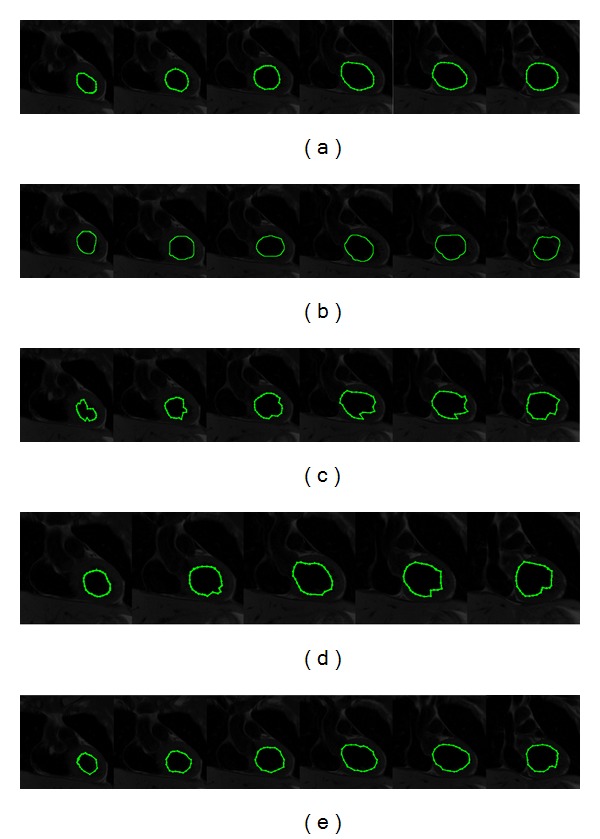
MR images (ventricular area segmentation): (a) manual delineation by experts, (b) results of Graph Cut method, (c) results of traditional ACM, (d) results of interactive Tseng method, and (e) results of proposed implementation.
